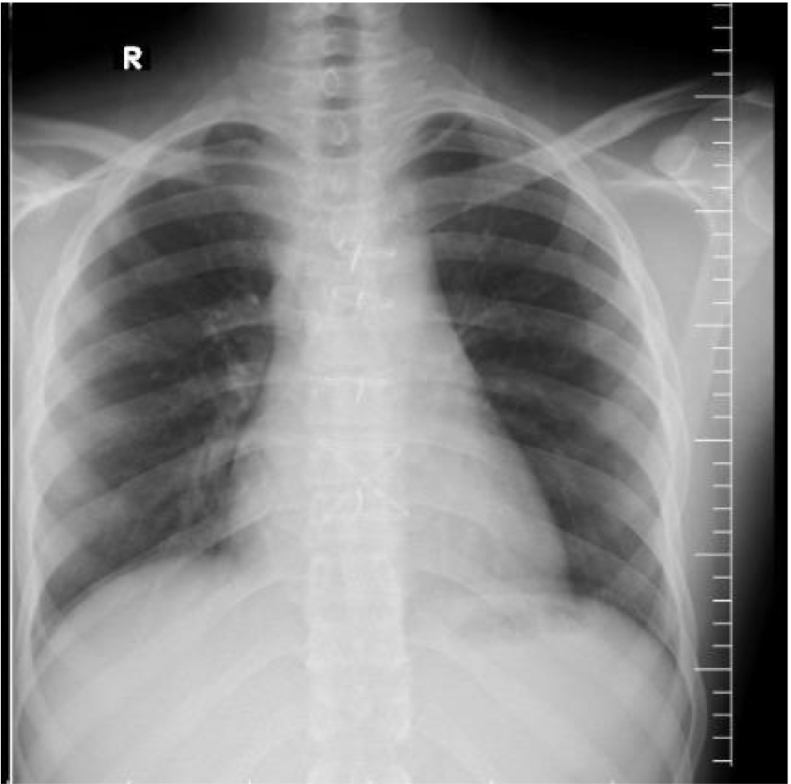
After 1 year of chest x-ray control.
